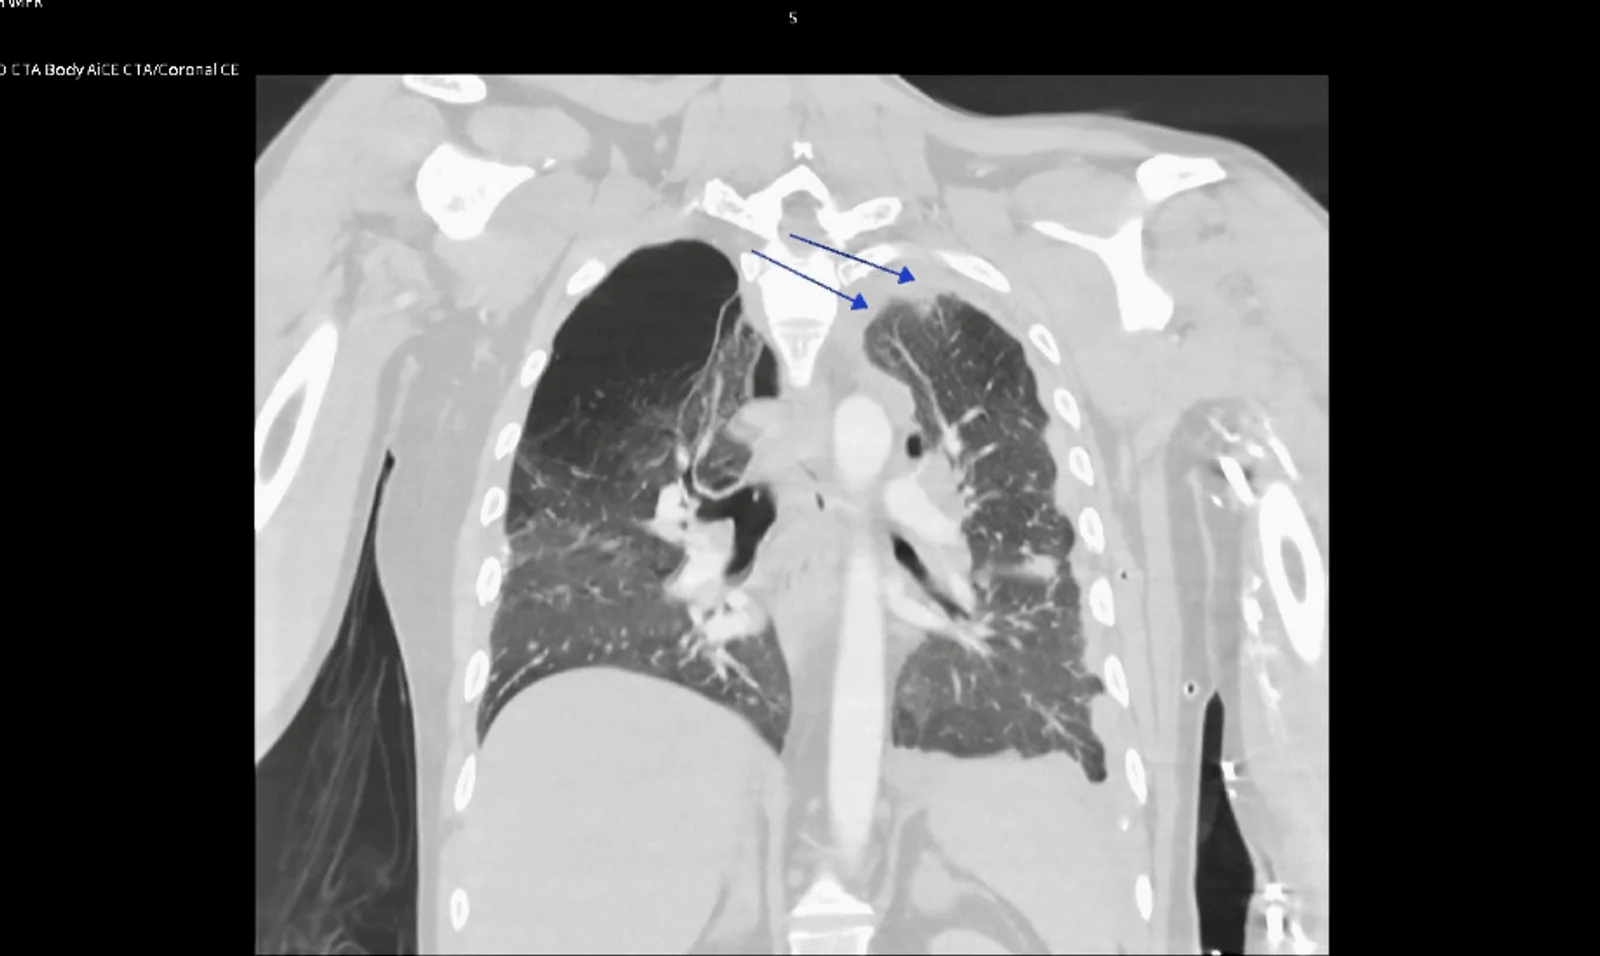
CTPA was negative for PE but showed established lung cancer (blue arrows) CTPA: Computed tomography pulmonary angiogram, PE: Pulmonary embolism
